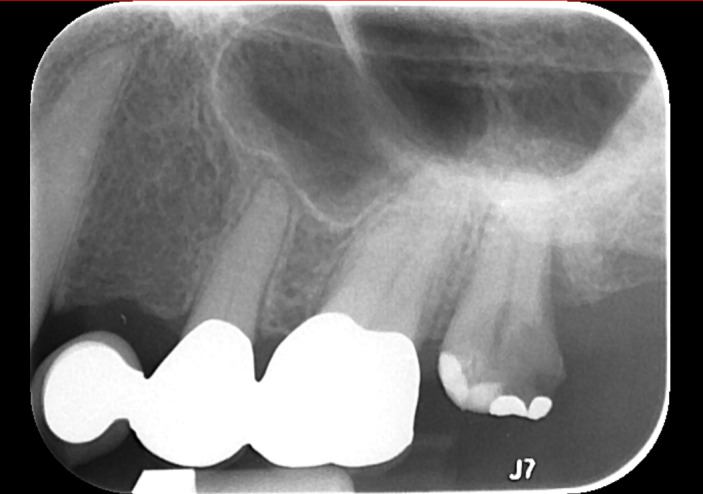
Periapical radiograph demonstrating a large carious lesion at no. 15 with associated widening of periodontal ligament and periapical radiolucency
